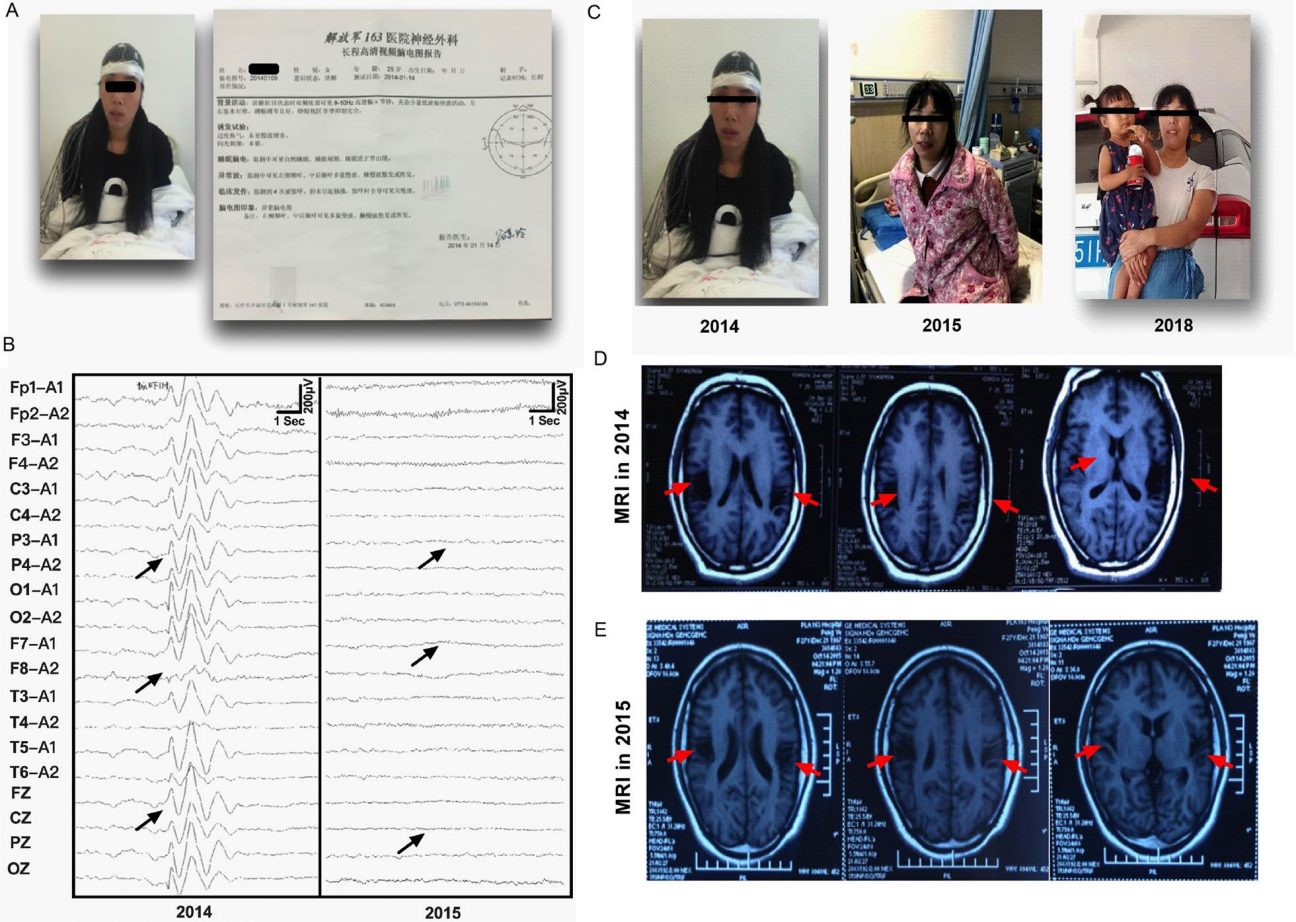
Clinical data of patient before and after autologous OM-MSC therapy. A A 26-year-old female suffered from chronic refractory seizures for 17 years. B The ambulatory electroencephalography (AEEG) was recorded the process of epileptogenesis in 2014 and 2015, before and after autologous OM-MSC therapy. C The clinical condition of patient by taking pictures in 2014 before autologous OM-MSC therapy, and follow-up in 2015 and 2018 after autologous OM-MSC therapy. D Brain magnetic resonance imaging (MRI) showed obviously focal malacia localizing in the bilateral temporal region as well as moderate generalized cerebral atrophy in 2012 before autologous OM-MSC therapy. E MRI showed no obviously changes in intracranial structure in 2015 relative to in 2014 (before OM-MSCs transplantation)
